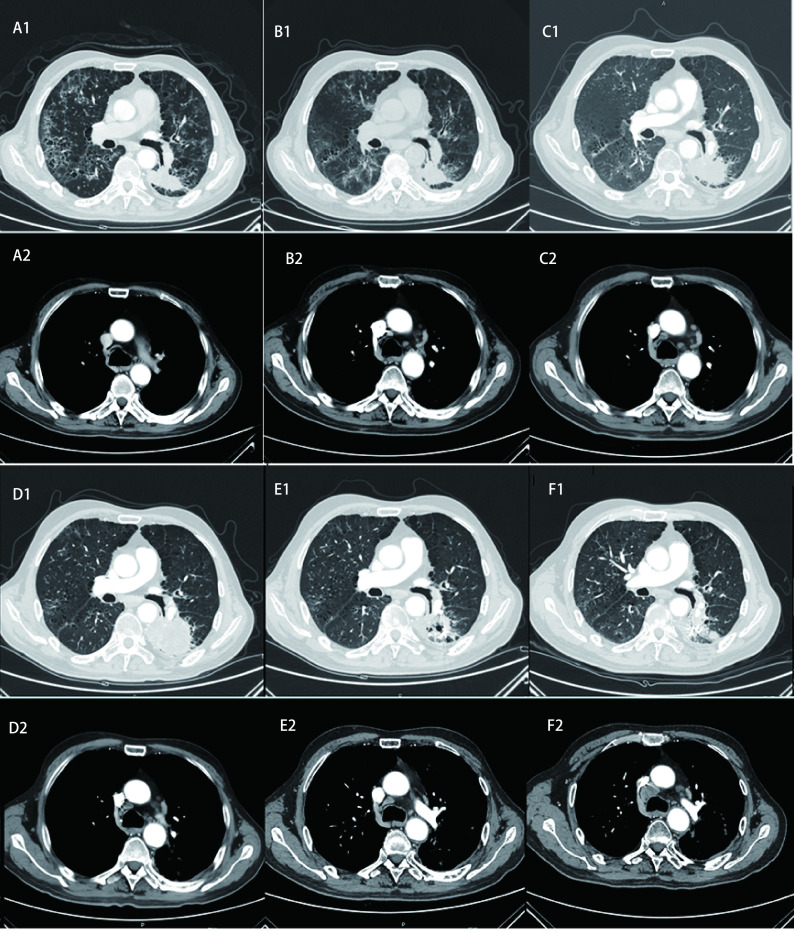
确诊CIP后抗肿瘤治疗CT疗效评价。A：白蛋白结合型紫杉醇化疗前基线CT（2019年11月18日）；B：白蛋白结合型紫杉醇化疗4个周期后CT（2020年1月16日），疗效评价SD；C：因疫情中断化疗2月后复查CT（2020年3月9日），肺部病灶进展；D：安罗替尼2个周期后CT（2020年5月14日），疗效评价SD（左下肺病灶略增大）；E：安罗替尼+左下肺病灶动脉栓塞术+碘125粒子植入术后CT（2020年7月14日），疗效评价PR；F：继续安罗替尼2个周期后CT（2020年9月22日），疗效评价PD（左下肺新发病灶，纵隔淋巴结增大）。 CT evaluation of anti-tumor therapy after diagnosis of CIP. A: Baseline CT before albumin binding paclitaxel chemotherapy (November 18, 2019); B: CT after 4 cycles of albumin binding paclitaxel chemotherapy (January 16, 2020). Efficacy evaluation was SD; C: CT scan after 2 months interruption of chemotherapy due to COVID-19 pandemic situation (March 9, 2020). The left lower lung lesion progressed; D: CT after 2 cycles of Anlotinib (May 14, 2020). Efficacy evaluation was SD (slightly enlarged left lower lung lesion); E: CT after Anlotinib+arterial embolization+iodine 125 seed implantation of left lower pulmonary lesion (July 14, 2020). Efficacy evaluation was PR; F: CT after sequential 2 cycles of Anlotinib (September 22, 2020). Efficacy evaluation was PD (newlesion in left lower lung, mediastinal lymph node enlargement). SD: stable disease; PD: progressive disease.
